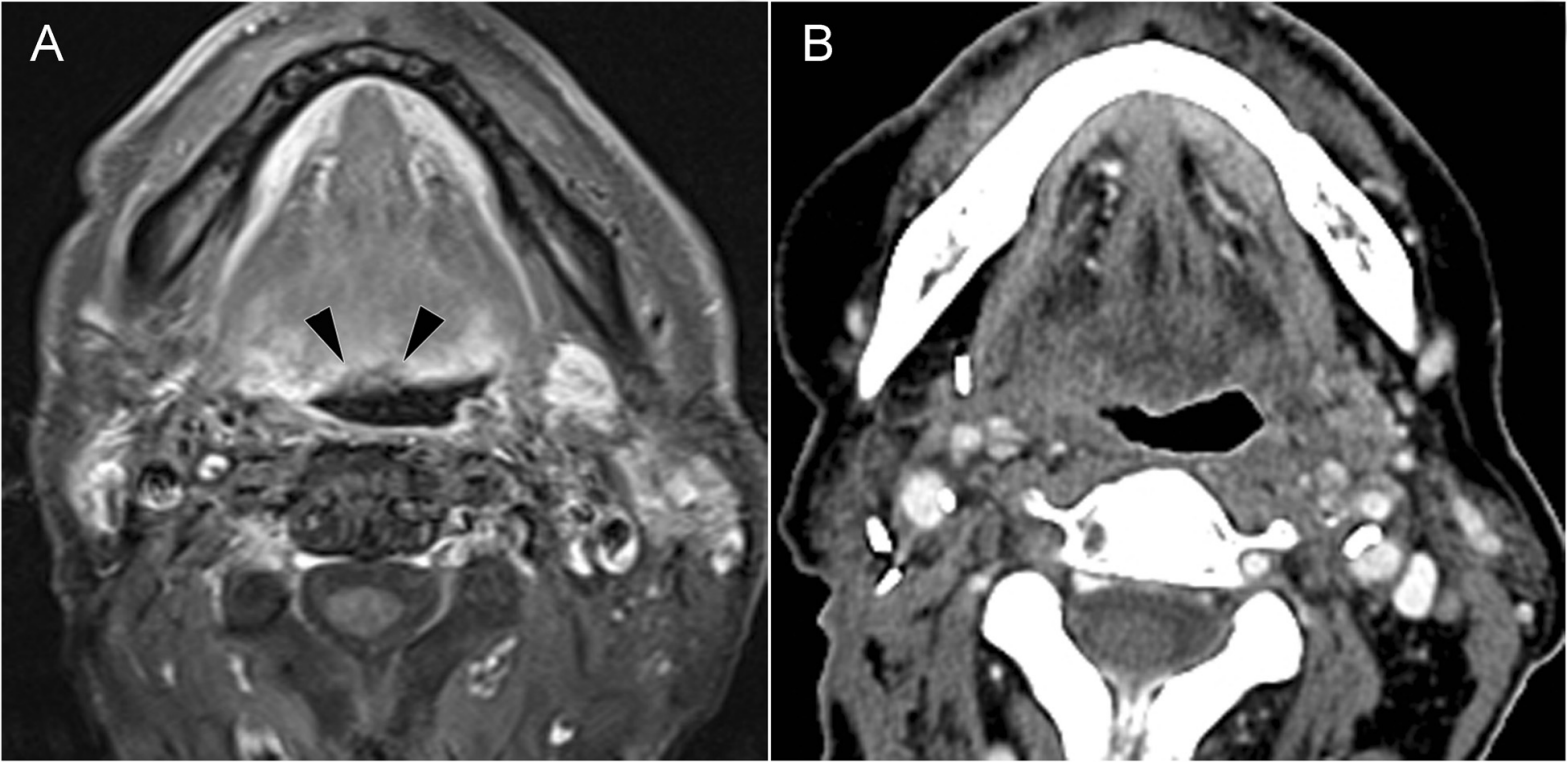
Patient 1. a Pretreatment axial contrast-enhanced fat-suppressed T1-weighed MR image shows small, irregular ulceration on the BOT midline. b Axial contrast-enhanced CT image: the BOT at 20 months post-TORS–post-PDT
